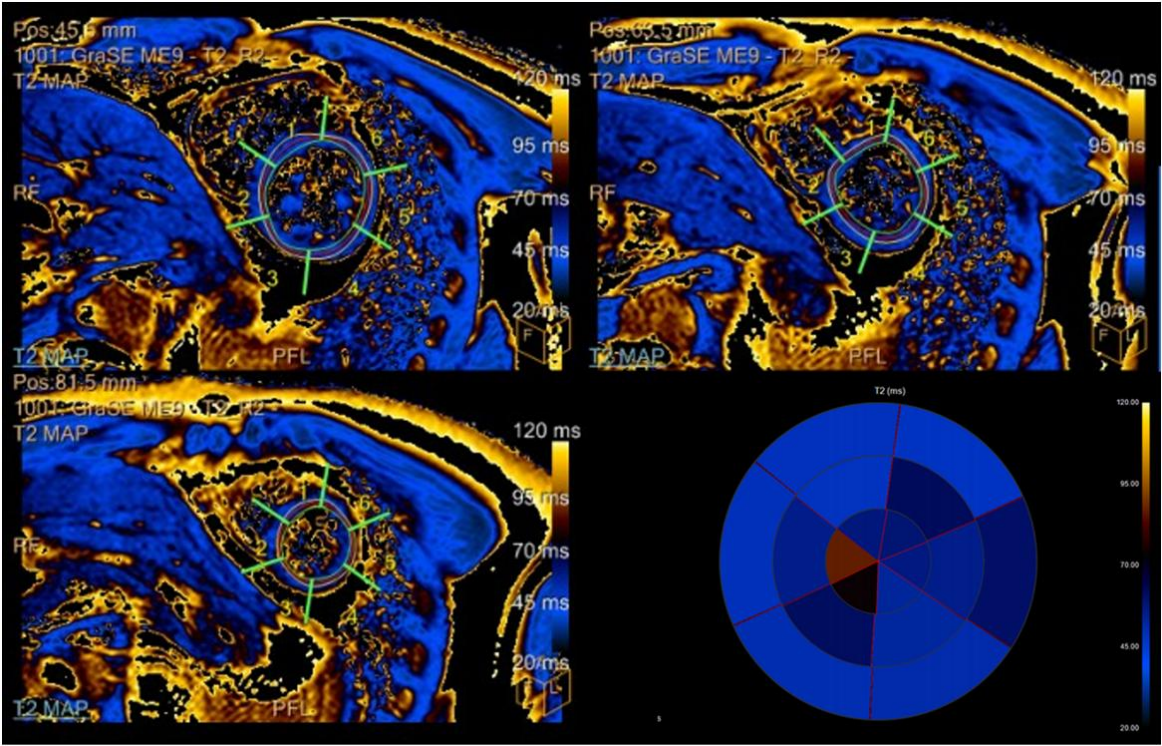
Cardiac MRI, T2-mapping sequence showing myocardial oedema.
